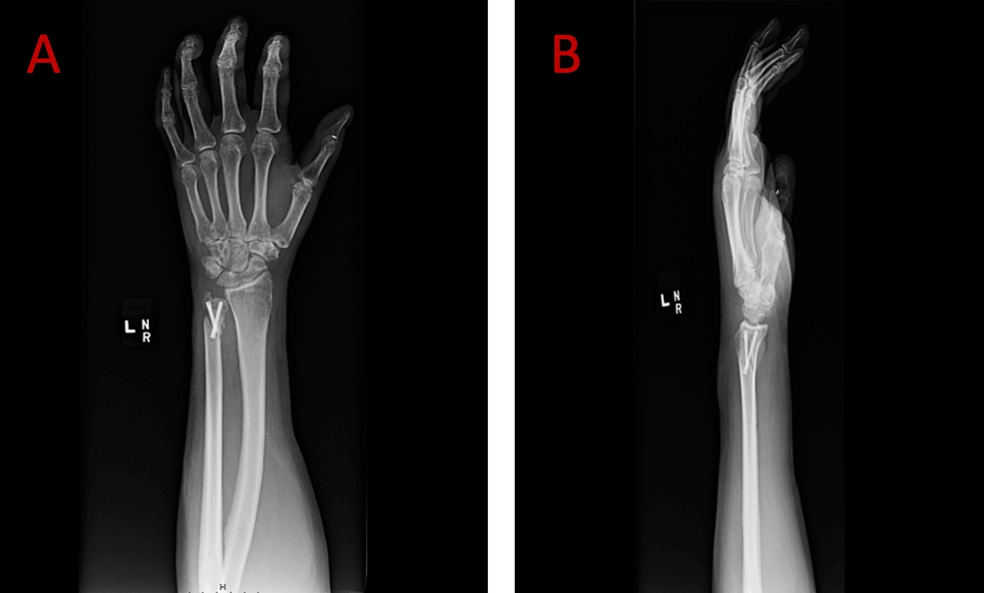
Anterior-posterior (A) and lateral (B) X-ray views of the left wrist at six weeks post distal ulna open reduction and internal fixation using headless compression screws.
